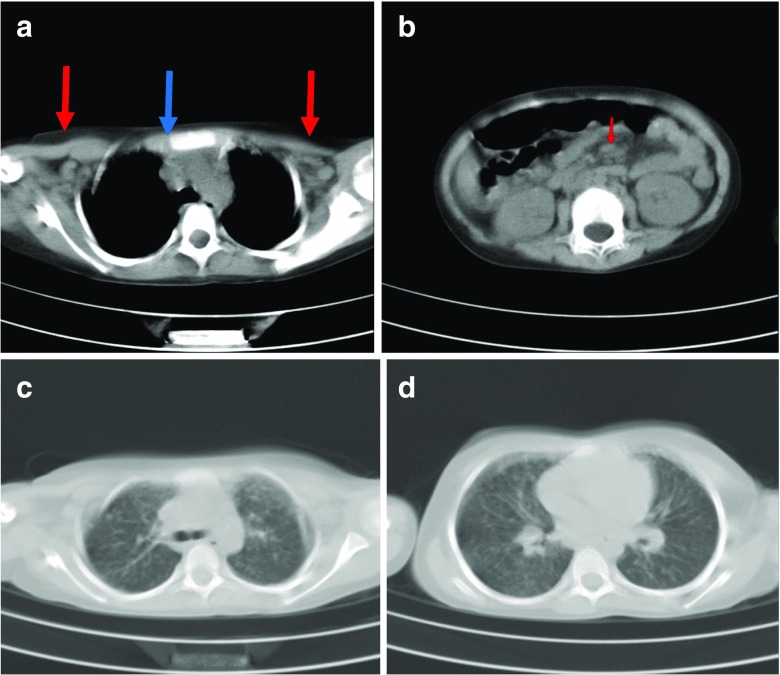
CT scan of lungs. a Axial chest CT scan (mediastinal window). Bilateral axillary (*red arrows*) and mediastinal lymphadenopathies (*blue arrow*). b Axial abdominal CT scan. Mesenteric lymphadenopathy (*red arrow*). c Axial chest CT scan without contrast. d Bilateral micronodular opacities along with reticulonodular infiltrations are seen
